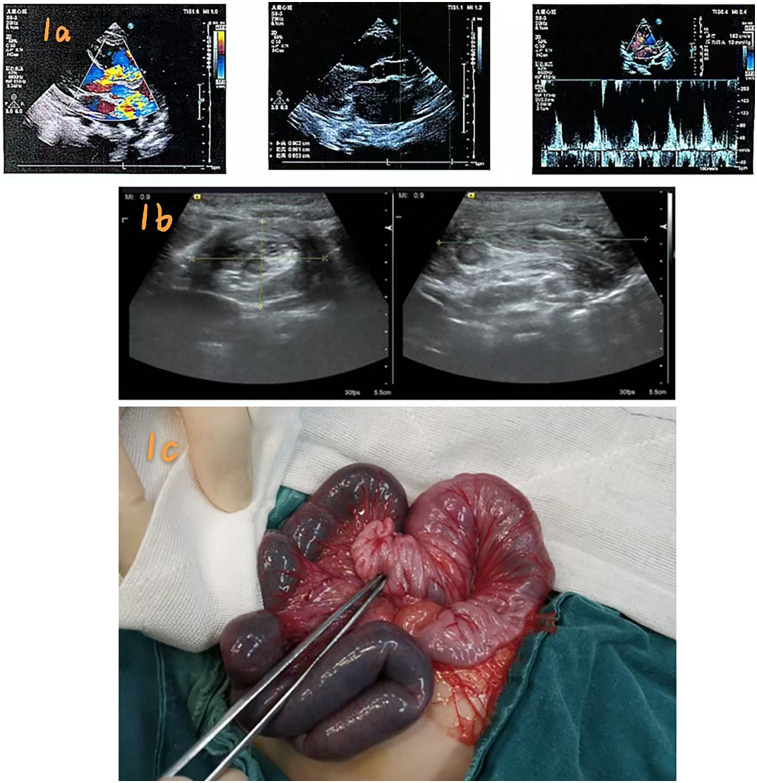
(a) Preoperative echocardiography findings; (b) Ultrasound “target sign” confirming ileocolic intussusception; (c) Intraoperative view showing ischemic ileum and intussuscepted segment.
